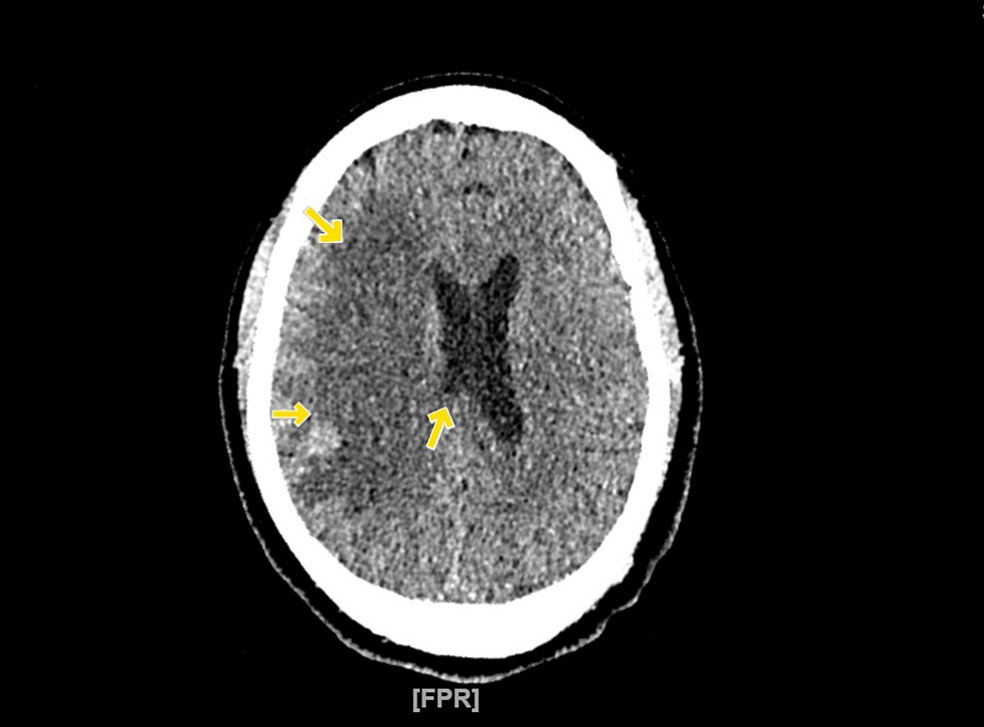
CT of the head without contrast Arrows show a large right MCA territory infarct. There is evidence of mass effect and midline shift from right to left measuring approximately 3.8 mm. CT: computed tomography, MCA: middle cerebral artery
